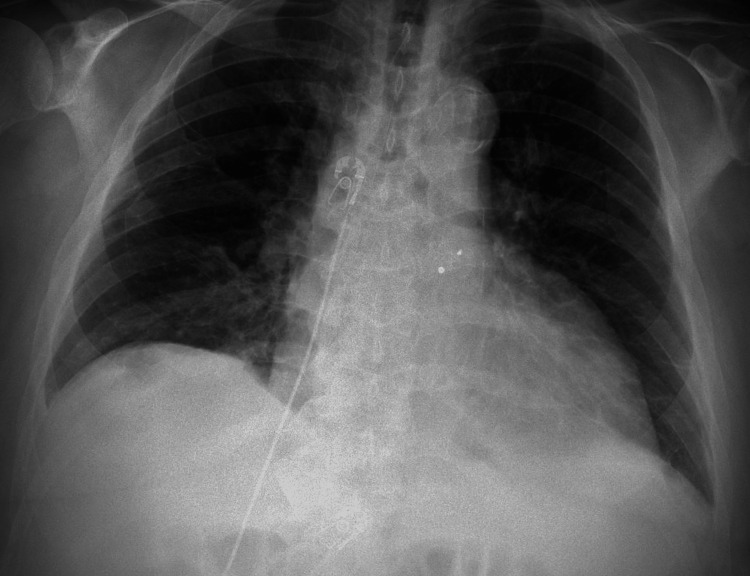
Chest X-ray showing cardiomegaly Official radiologist final impression: No acute process. Cardiomegaly.
